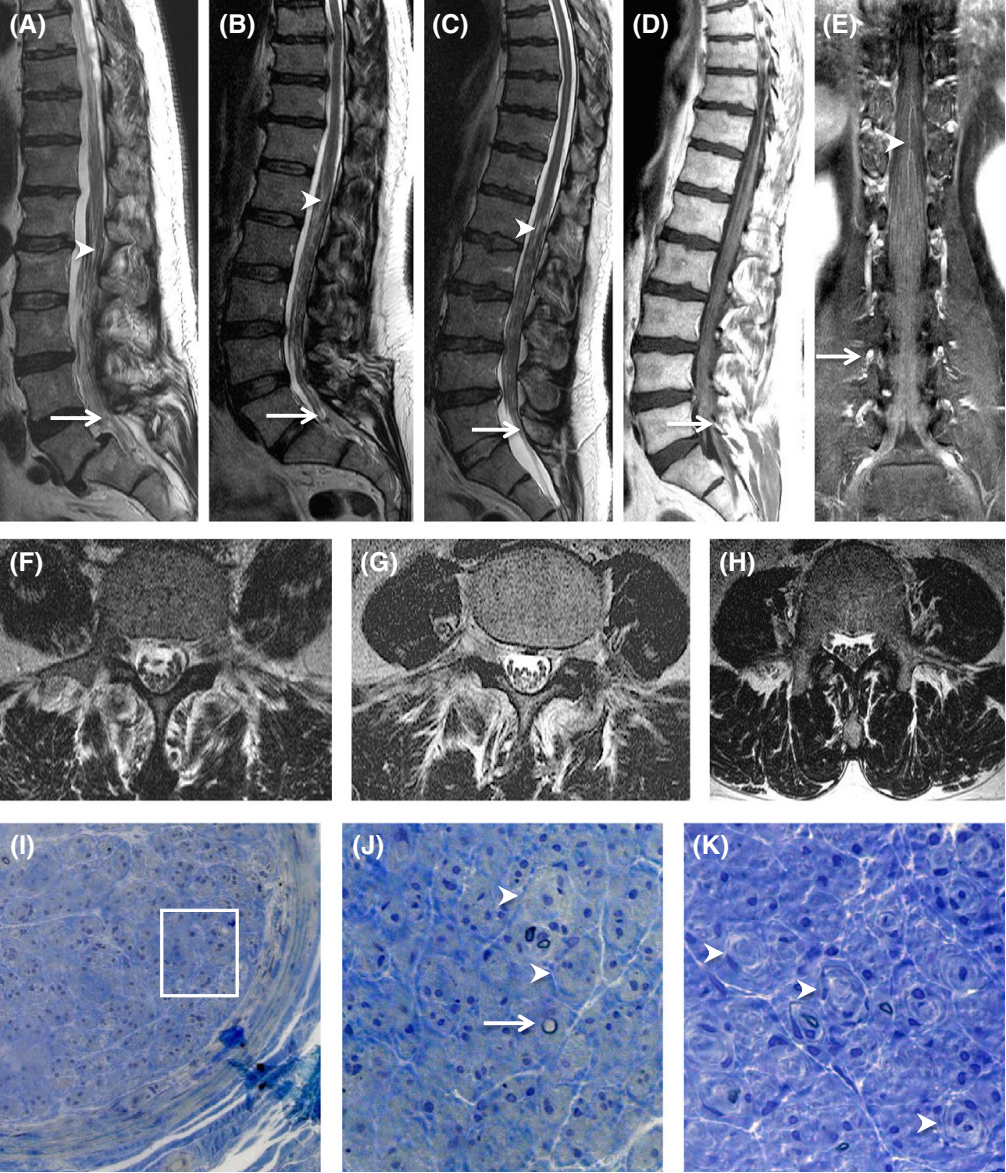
Thoraco‐lumbo‐sacral MRI and sural nerve biopsies in three CMT1B patients with *MPZ*‐mutation and hypertrophic cauda equina/nerve roots. (A–C) T2‐weighted sagittal sections of the thoracal, lumbar, and sacral spinal column in patients 6 (A), 4 (B), and 2 (C), indicating the thickened nerve roots of the cauda equina (arrows). This is also shown for patient 2 at a T1‐weighted sagittal section (D) and a T1‐weighted coronal section (E). Arrow heads show congested intraspinal vessels. (F–H) T2‐weighted axial sections at the level of the fourth lumbar vertebral body (L4) of patients 6 (F), 4 (G) and 2 (H), showing extensive hypertrophic nerve roots of the cauda equina inside the spinal column. (I–K) Nerve Biopsy pictures of patients 6 (I, J) and 2 (K) with (J) being a magnification of (I). Arrow heads show onion bulb formations, the arrow points toward a remaining hypomyelinated nerve fiber. Notice the extensive loss of the large myelinated fibers.
